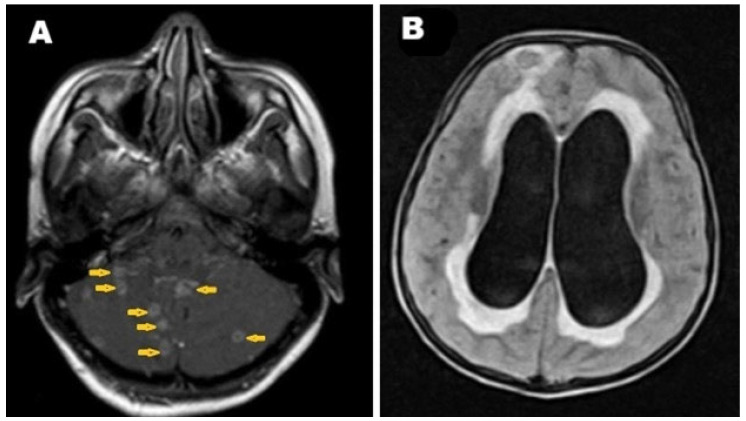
Selected brain MRI images. (A) Post-contrast T1-weighted image shows multiple intracranial ring-enhanced lesions (arrows) involving the cerebellum, strongly suggesting tuberculous foci, with perifocal edema causing mass effect upon the fourth ventricle. (B) FLAIR-weighted image shows obstructed hydrocephalus with active permeation appearing as a high signal intensity around the ventricles.
